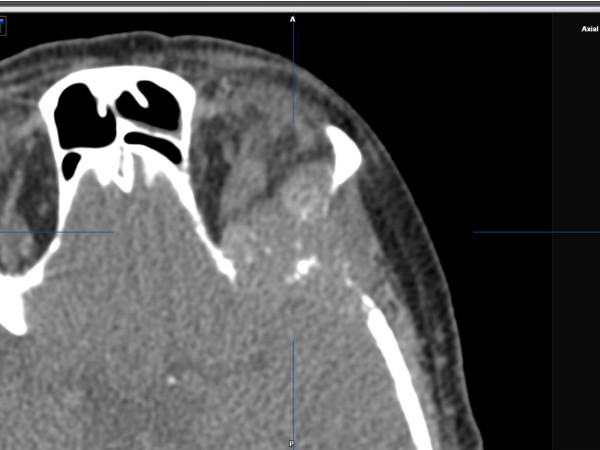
The axial CT scan shows an infiltrating tumor mass with bony destruction of lateral orbital wall and skullbase.
